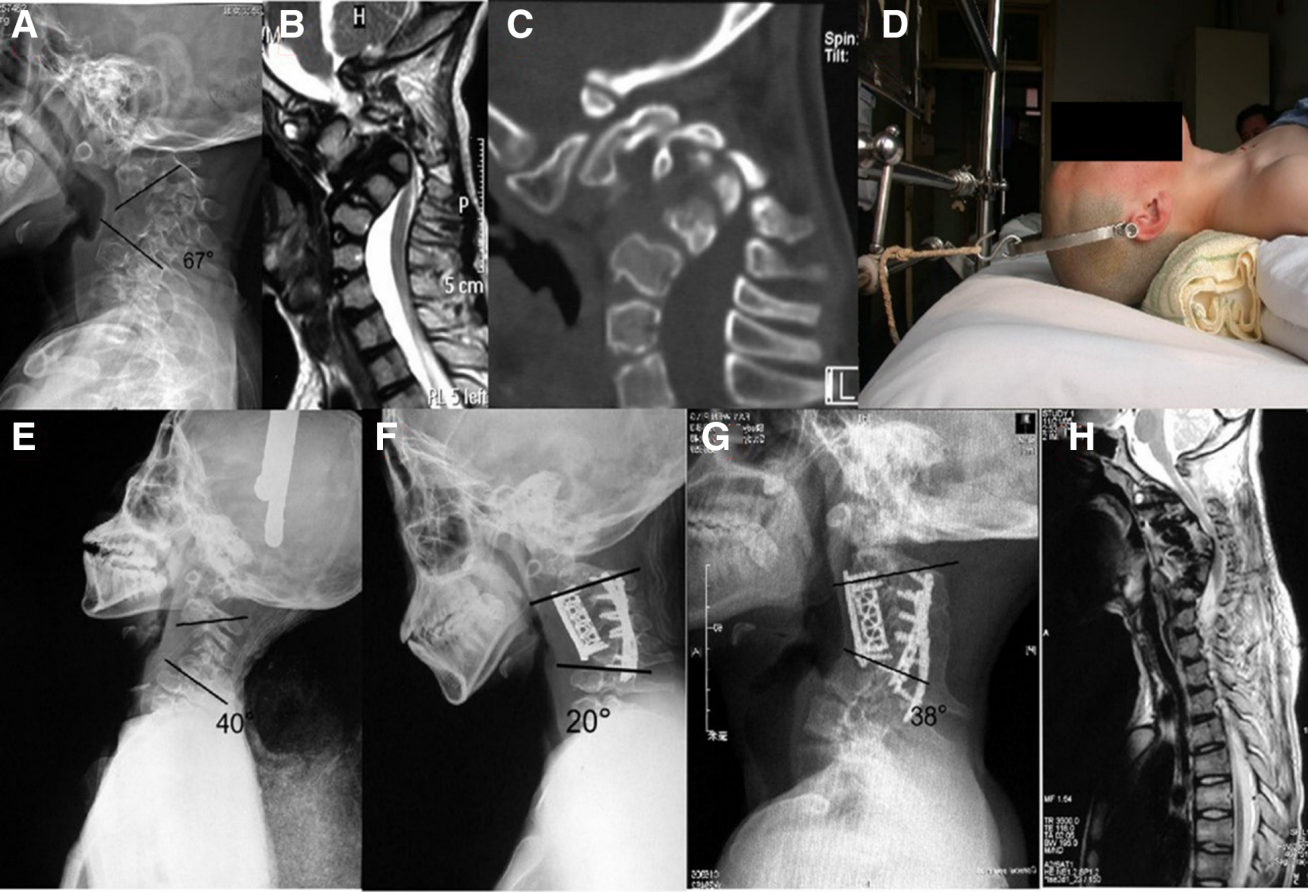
Case 1. This 15-year-old male complained of tetraplegia caused by osteochondrodysplasic cervical kyphotic deformity. (A) Pre-operative x-ray revealed a C2–7 Cobb angle of 67° before traction. (B) A para-sagittal computed tomography (CT) reconstruction showed no facet joint fusion. (C) Sagittal magnetic resonance imaging (MRI) showed severe compression of spinal cord at C3–5 level. (D) Skull bone traction. (E) Two weeks after skull bone traction, the Cobb angle had reduced to 40°; the correction rate was 40.3%. (F) Post-operative x-ray showed the Cobb angle reduced to 20°. (G) x-ray at 4 years showed progression of kyphosis at C6–7 level. (H) MRI at 4 years showed incomplete decompression of cervical spinal cord with Japanese Orthopedic Association scale (mJOA) score improved from 11 to 13 post-operatively.
